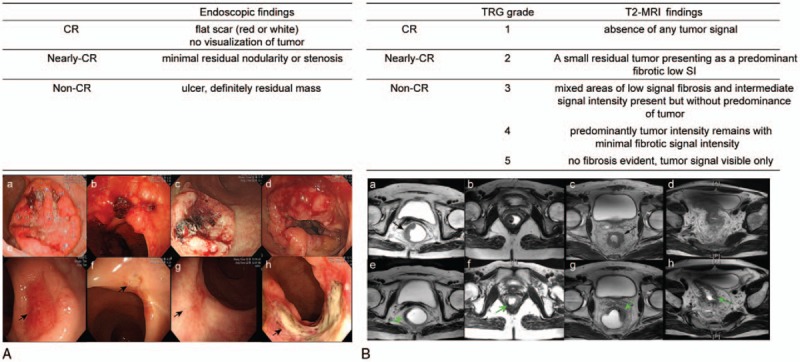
(A) Tumor on endoscopy before and after preoperative chemoradiation therapy (pre-CRT). (a–d) Primary tumor before pre-CRT. (e, f) Clinically complete response of primary tumor after completion of pre-CRT; a typical sign of clinical complete response (black arrows indicate a red scar and a white scar). (g) Minimal residual nodularity and (h) residual tumor with large necrotic area after pre-CRT (a typical sign of poor tumor response). (B) Magnetic resonance imaging (MRI) scans from patients before and after pre-CRT. (a–d) Primary tumor before pre-CRT. (e) Tumor regression grade (TRG) 1; the green arrow indicates radiologic complete response with a hypointense rectal wall. (f) TRG 2; the green arrow indicates a slightly thickened hypointense rectal wall. (g) TRG 3; the green arrow indicates a visible intermediate signal. (h) TRG 4; the green arrow indicates a small area of fibrosis.
